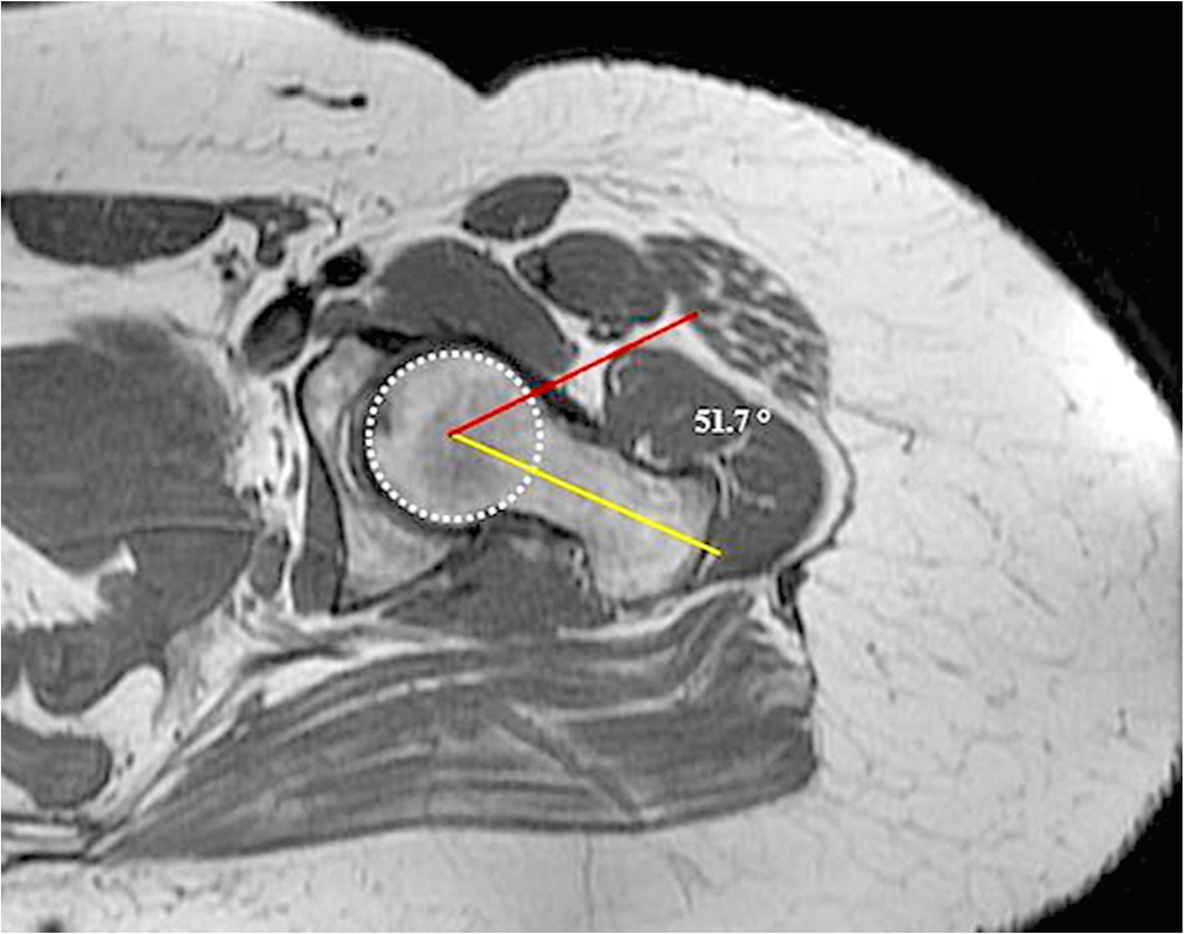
Measurement of alpha angle on MRI view of the left hip of a 24-year-old woman (the *yellow line* extends from the center of the femoral head to the midpoint of the femoral neck; the *red line* is extended from the center of the femoral head to the deviation of the femoral neck from the *circle* drawn around the femoral head)
